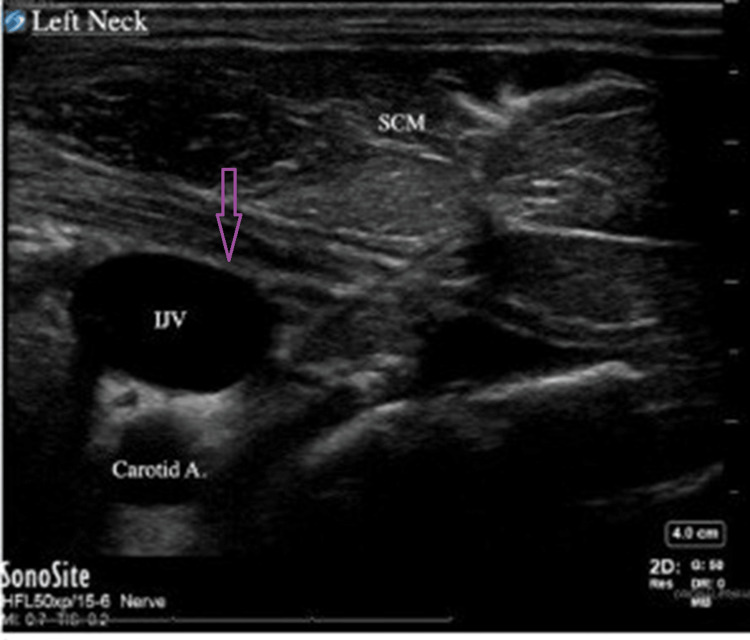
Ultrasound image of the left side of the neck showing purple arrow directed to IJV IJV: internal jugular vein
